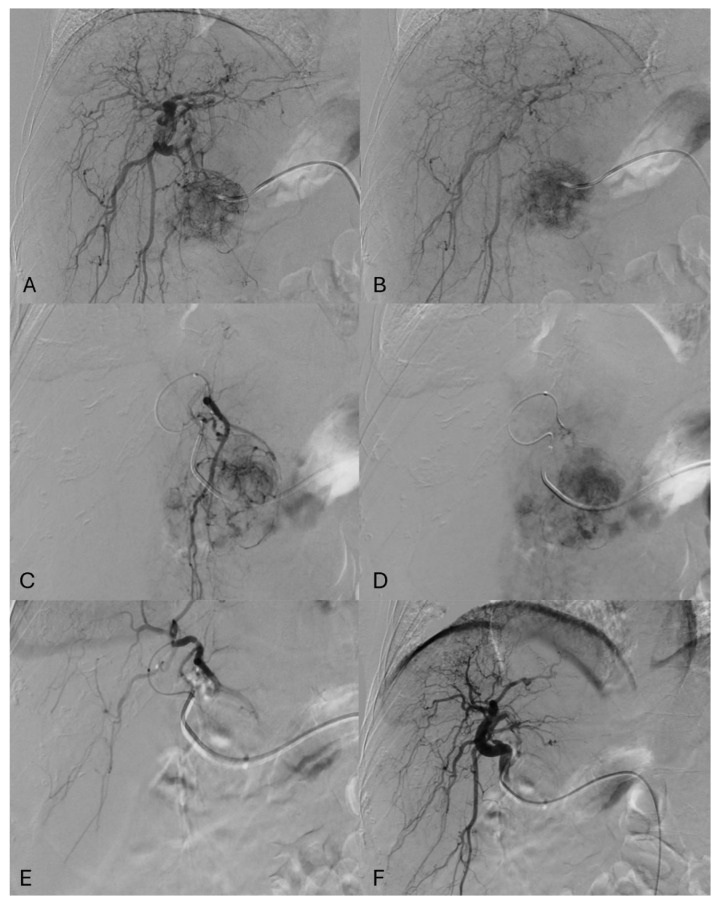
(A) Digital subtraction angiography (DSA), performed with Cobra C2 catheter tip (Imager II, Boston Scientific Corporation, Marlborough, MA, USA) positioned inside the proper hepatic artery with automatic injector (12 mL, 3 mL/s, 700psi, Iomeron 350, Bracco, IT), shows hepatic arterialization with anarchic hepatic nodule hypervascularization with characteristic “basket sign” pattern. (B) DSA at late arterial phase shows wash-in with retention of contrast media inside the hepatic nodule. (C,D) DSA shows superselective angiograms obtained using a microcatheter (Progreat 2.4, Terumo Inc., Tokyo, Japan). Here, each vessel supplying the HCC nodule is selectively catheterized, and microspheres (Embogold 100–300 micron, MERIT Medical System, South Jordan, UT, USA) are used to obstruct (embolize) these arteries. (E) shows a DSA from microcatheter demonstrating minimal to no blood flow in feeders vessels of HCC nodule. (F) the final DSA performed with the tip of the Cobra C2 catheter at the level of the proper hepatic artery confirms the procedure’s success by showing effective embolization as no contrast media reaches the anarchic vasculature of the HCC nodule. Following TAE or MWA, patients undergo meticulous monitoring to ensure successful outcomes. This typically involves a structured post-procedural care plan. Blood tests repeated 24 h and 72 h after the procedure check for abnormalities in blood cell counts, liver function, and kidney function—potential indicators of complications. Outpatient clinic visits are scheduled regularly, often starting one month after the procedure. At one month, blood tests and MRI are performed to evaluate the treated area and assess treatment tumor response.
